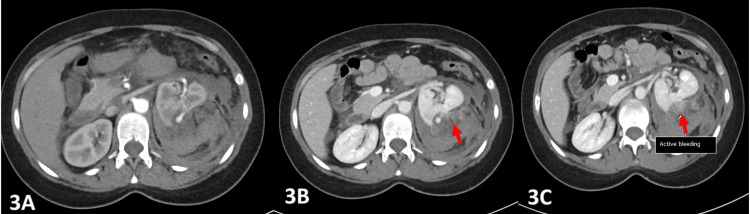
Selected contrast-enhanced axial CT images (arterial phase, 3A; venous phase, 3B; and delayed phase, 3C) demonstrate active contrast extravasation (arrow) and a well-defined saccular lesion in the upper posterior kidney. The lesion follows an arterial enhancement pattern, with avid enhancement in the arterial phase, slight increase in the venous phase, and washout on delayed imaging.
